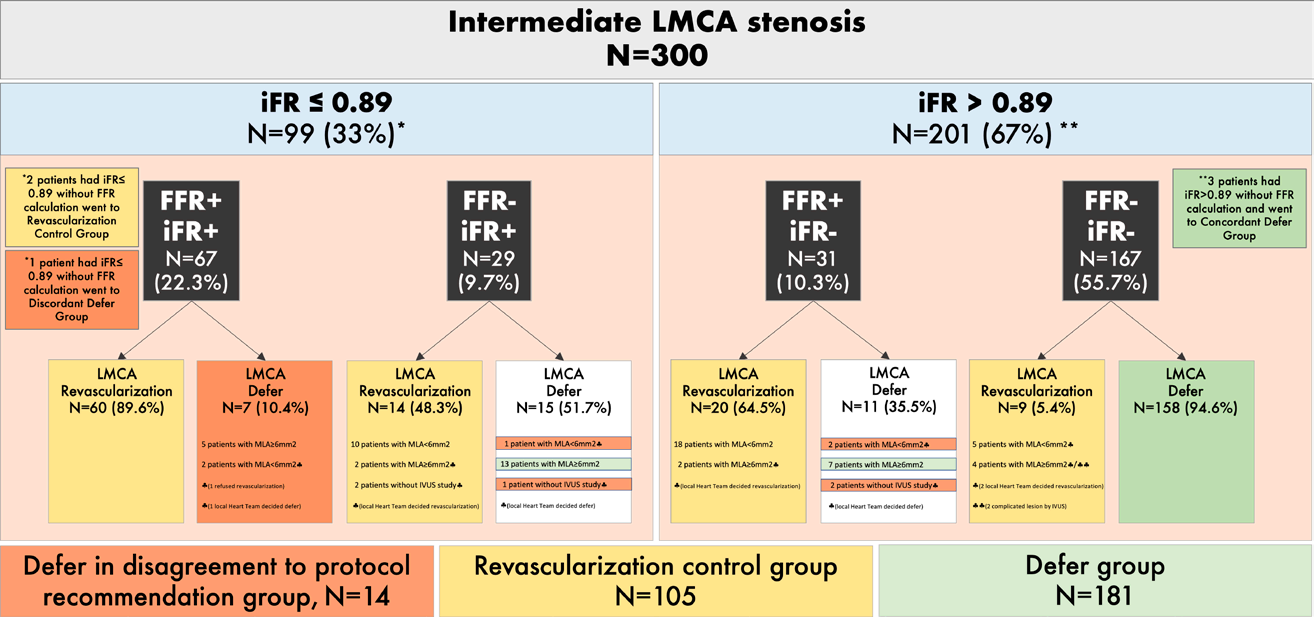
Treatment decision according to instantaneous wave-free ratio (iFR), fractional flow reserve (FFR), and minimal lumen area (MLA) values. In patients with discordant FFR and iFR, protocol recommended to perform intravascular ultrasound (IVUS) and decide revascularization if MLA<6 mm^2^, but local heart team had the final decision. LMCA indicates left main coronary artery.
